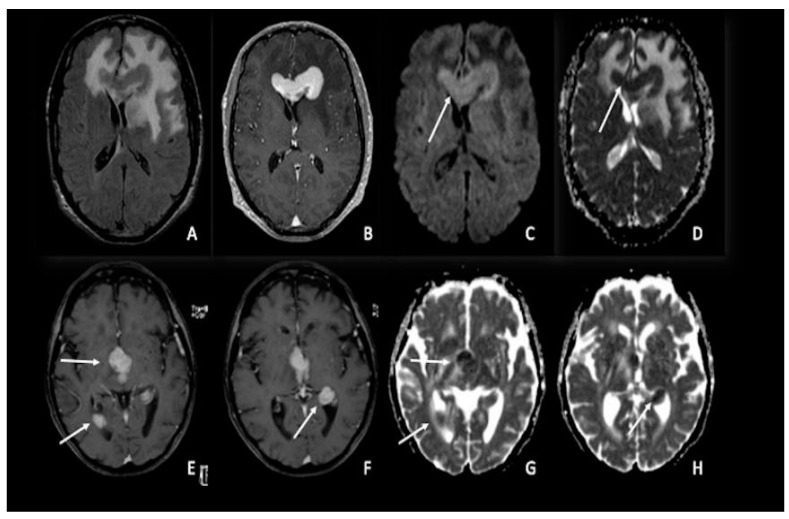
Two different cases of primitive cerebral lymphoma: the upper row showed a lymphoma in the pericallosal region (A–D) with a marked uniform enhancement on post-contrastographic T1 weighted image (B) and significant edema in the adjacent brain (A). The lower row showed a periventricular multicentric lymphoma (E–H) with multiple nodules of marked enhancement on post-contrast T1 weighted images (arrows) (E,F). Both cases presented restricted water diffusion with hyperintensity on DWI (arrow in C) and hypointense areas on ADC (arrows respectively in (D,G,H)), related to increasing tumor cellularity, as occurs typically in lymphomas. DWI = diffusion-weighted imaging; ADC = apparent diffusion coefficient.
